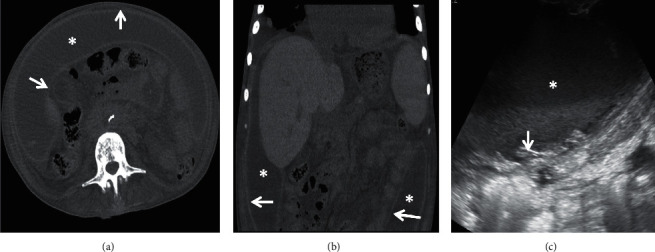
A 52-year-old male with sclerosing encapsulating peritonitis. (a) Axial noncontrast CT of the abdomen showing encapsulated ascites (∗) within the thickened layers of the peritoneum (arrows). The bowel loops are displaced centrally due to the mass effect. (b) Coronal noncontrast CT image shows the extent of the encapsulated fluid (∗) within the thickened peritoneum (arrows). (c) Grayscale ultrasound image acquired just prior to the paracentesis shows a low level of homogenous echotexture of the ascitic fluid (∗) and encapsulating thickened peritoneum (arrow).
